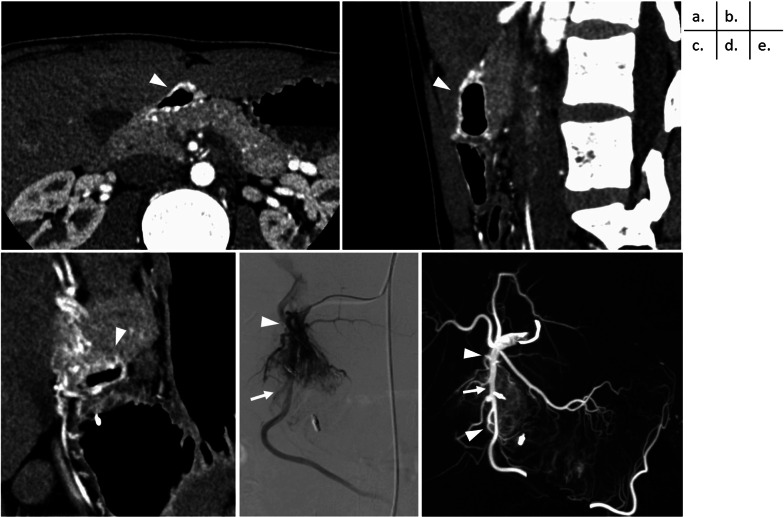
Preoperative contrast-enhanced computed tomography (CECT) and angio-CT images. a–c CECT images showing a hyper-enhancing duodenal bulb wall (arrowhead). No nidus is found in the pancreatic head. (a cross section, b sagittal section, c coronal section). d Angiography shows the arteries flowing into the duodenal AVM [arrow: inferior pyloric artery (IPA), arrowhead: superior duodenal artery (SDA)]. e Angio-CT images also show that the inferior pyloric artery (IPA) and superior duodenal artery (SDA) branching off from the gastroduodenal artery (GDA) are the inflow vessels. (arrow: GDA; arrowhead: IPA and SDA)
